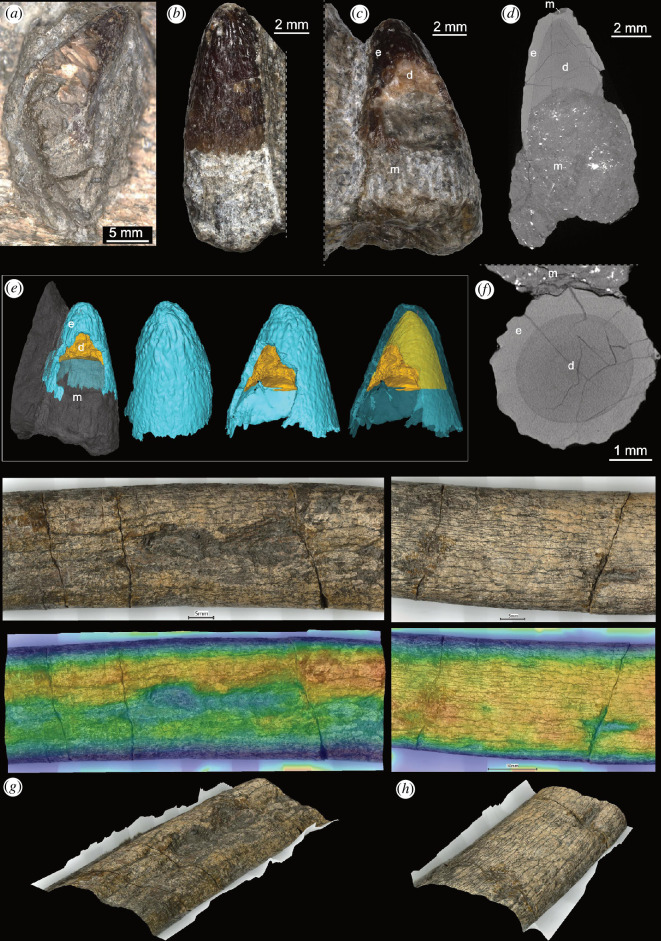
Photographs, CT scan and three-dimensional images of Swiss Machimosaurini tooth crowns and selected scavenging traces on the ichthyosaur ribs (PIMUZ A/III 5279). (*a*) Tooth crown PIMUZ A/III 5281b on left angular. (*b*,*c*) Tooth crown PIMUZ A/III 5281c on the axis neural arch. (*d*,*f*) CT scan images showing longitudinal and horizontal sections of PIMUZ A/III 5281c. (*e*) Three-dimensional model of PIMUZ A/III 5281c in different views, not to scale. (*g*,*h*) Normal, false colour and angled views of the ribs showing bite marks. Abbreviations: d, dentine; e, enamel; m, sediment matrix.
